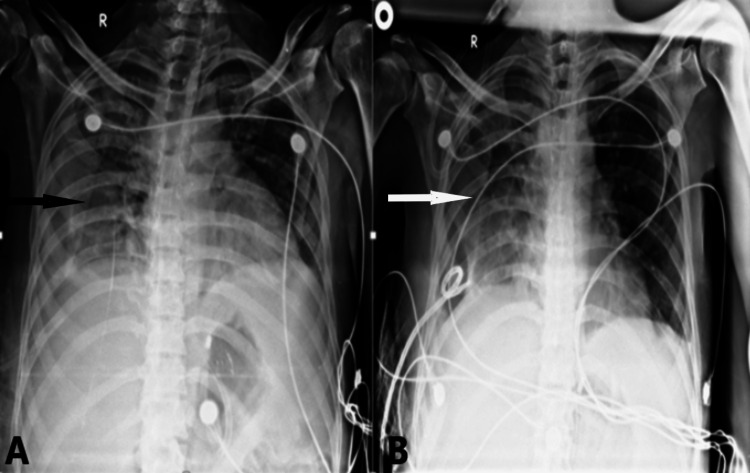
Case 5 A 50-year-old COVID-19 positive came with complaints of fever and breathlessness for three days. SpO2 on admission was 75%. (A) On admission chest radiograph, black arrow shows diffuse airspace opacities involving right lung parenchyma suggestive of consolidatory changes. (B) Follow-up X-ray after two days showed a resolution of consolidatory changes indicated by the white arrow. The patient was discharged five days later.
